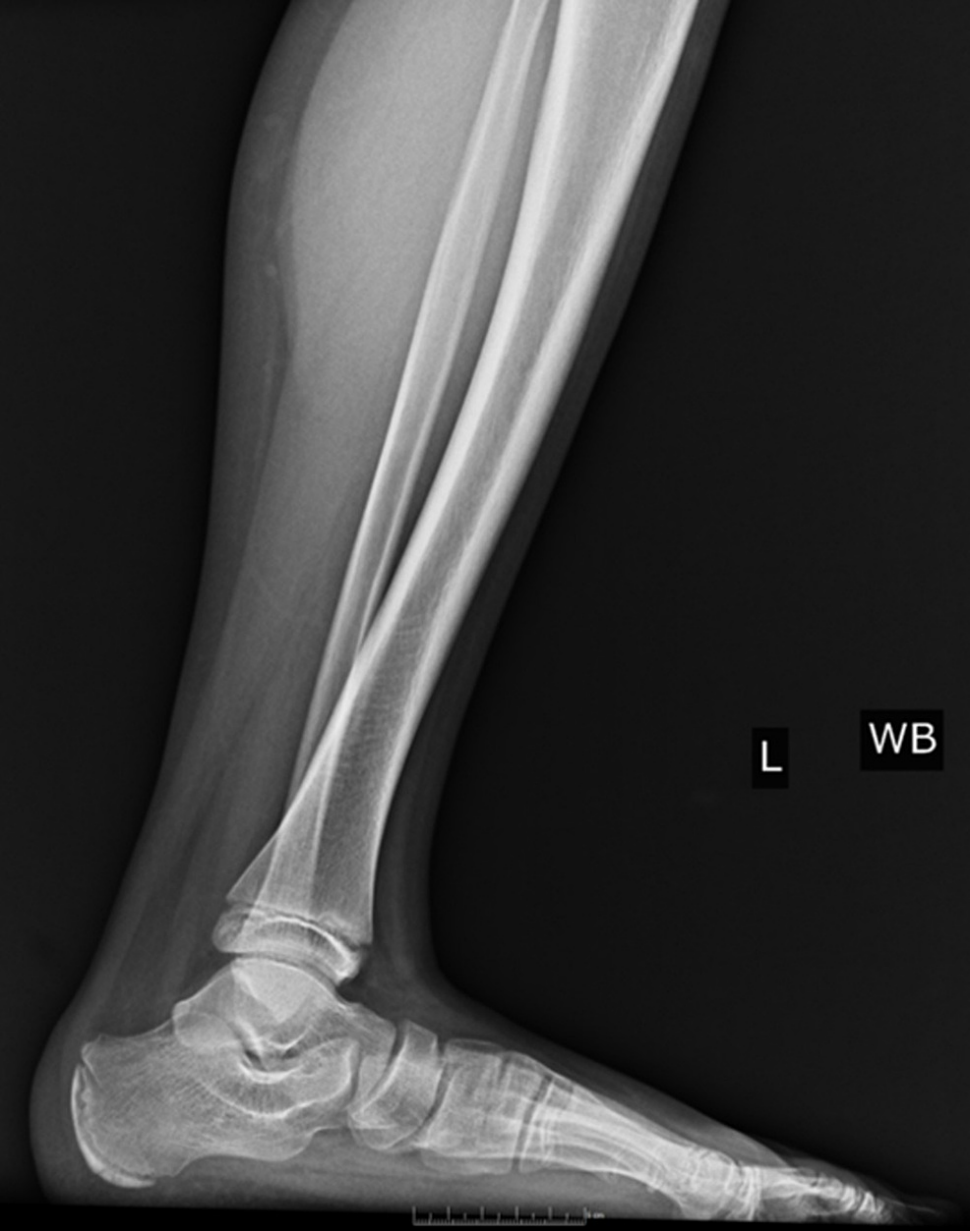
Lateral preoperative left foot weight-bearing X-ray.
